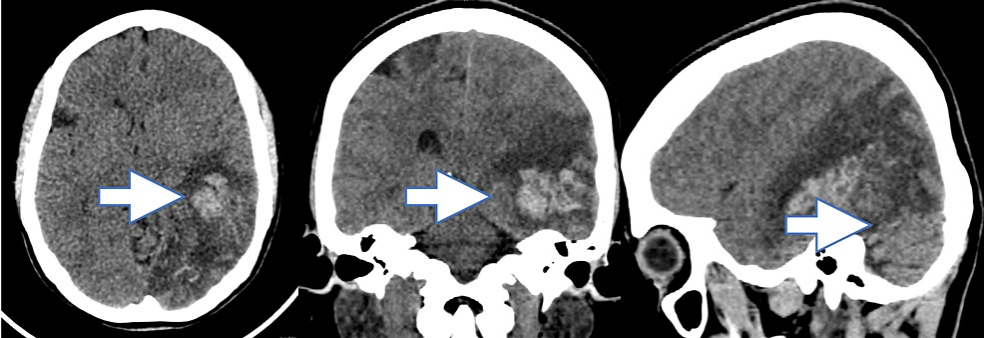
CT Brain showing intraparenchymal hemorrhage in parietal (axial), temporal (coronal) and occipetal (sagittal) views.
